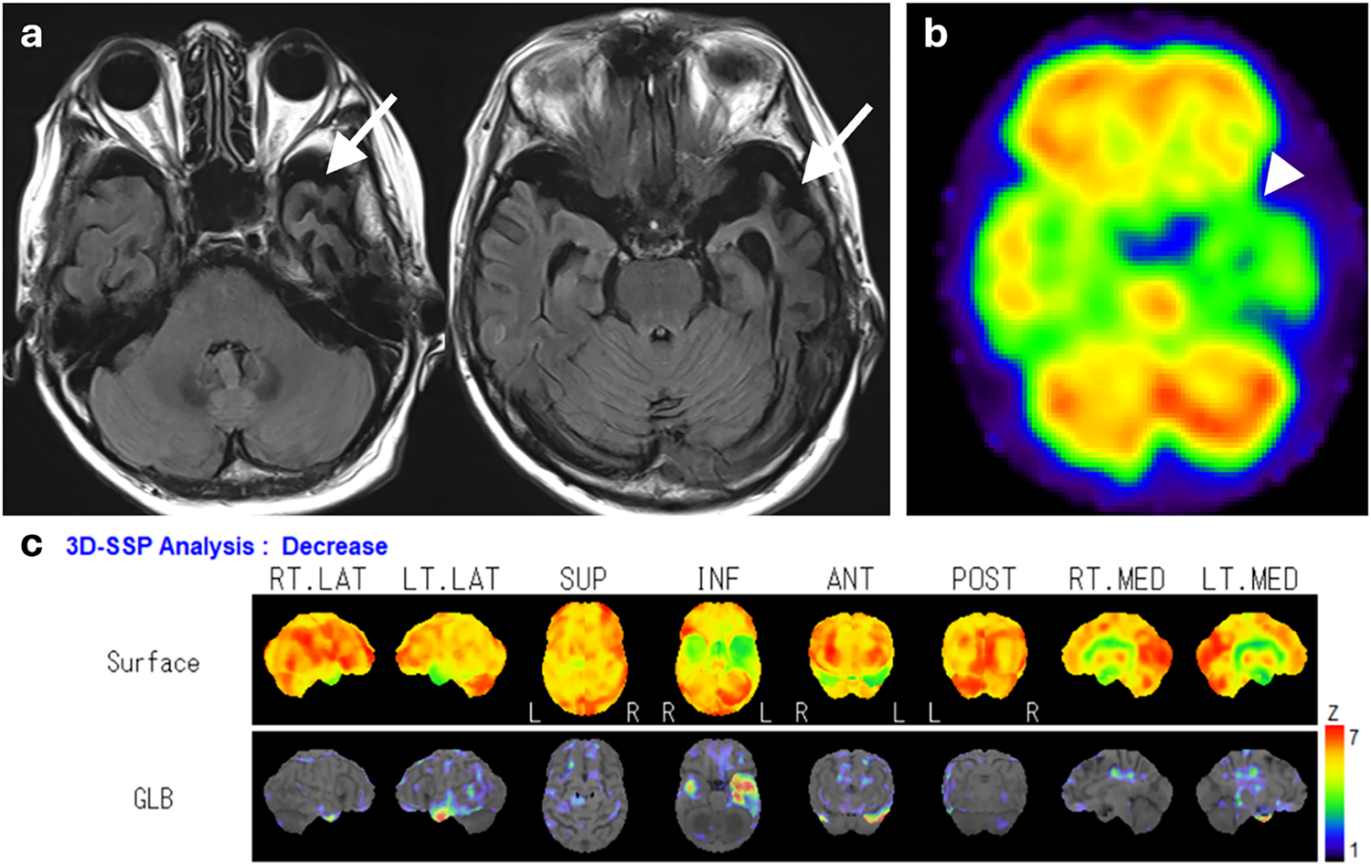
A 60-year-old female with SD. a Axial FLAIR images displayed atrophy in the anterior left temporal lobe (arrows), and b IMP brain perfusion images (arrowhead) and c 3D-SSP analysis revealed hypoperfusion in the same area, consistent with SD
